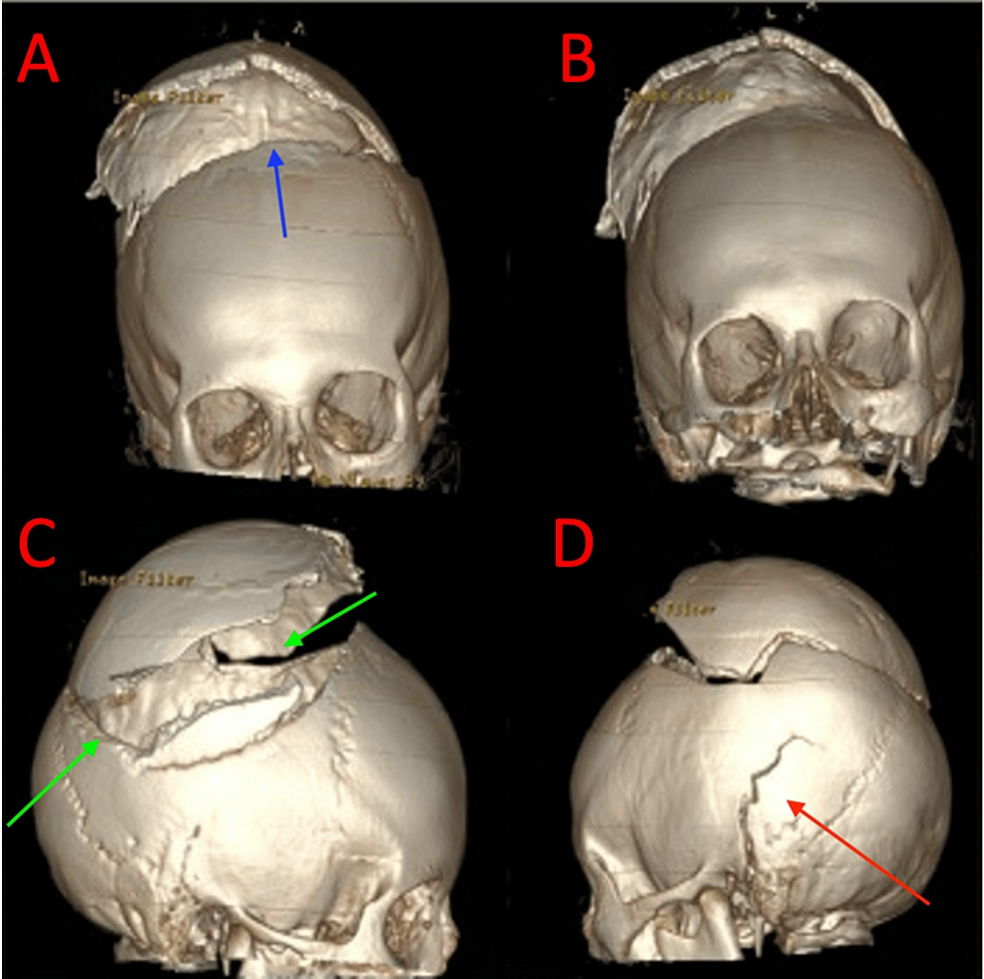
Extensive open skull fractures. Computed tomography volume-rendered three-dimensional (3-D) reformats demonstrating comminuted, displaced, open calvarial fractures involving the bilateral temporal (red arrow) and parietal bones (green arrows), as well as the frontal bone (blue arrow).
